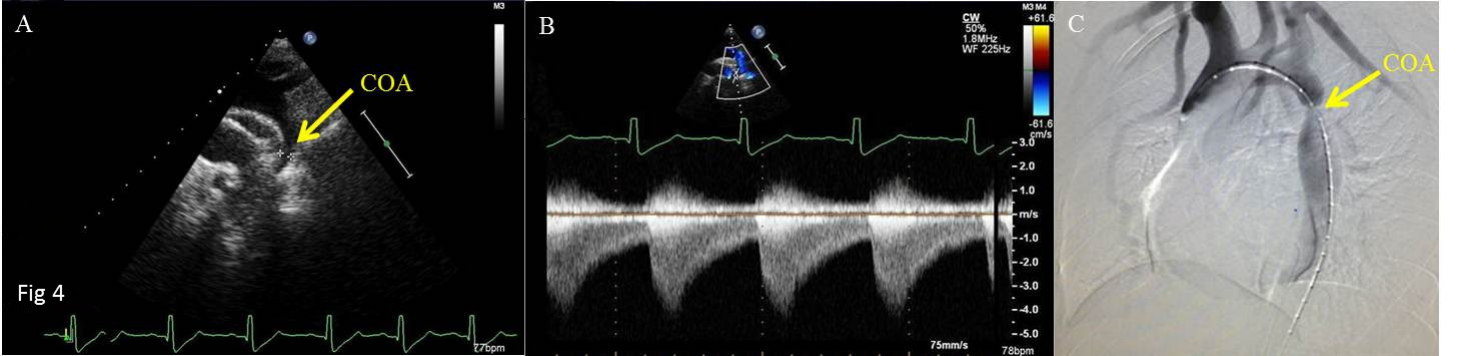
Transthoracic echocardiograms in suprasternal view of a19-year-old boy showing [A] the location of the CoA and [B]characteristics of the CoA by continuous wave Doppler, including high speed jet spectrum during systole and extended speed reduction during diastole. [C] Aortic angiography showed the location of the CoA. Abbreviations as in Fig 2.
